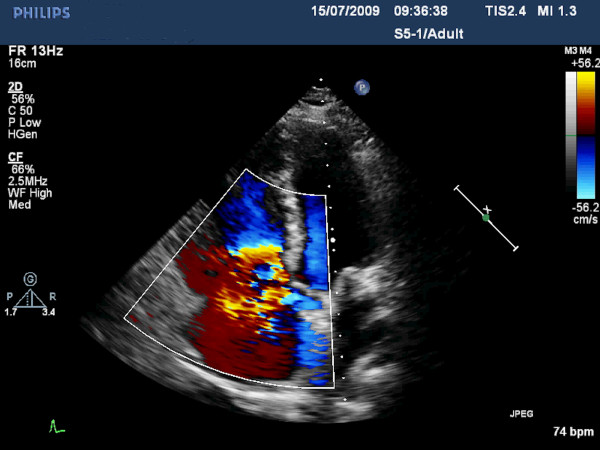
Apical four-chamber view in diastole. The color Doppler demonstrates severe tricuspid valve regurgitation.
